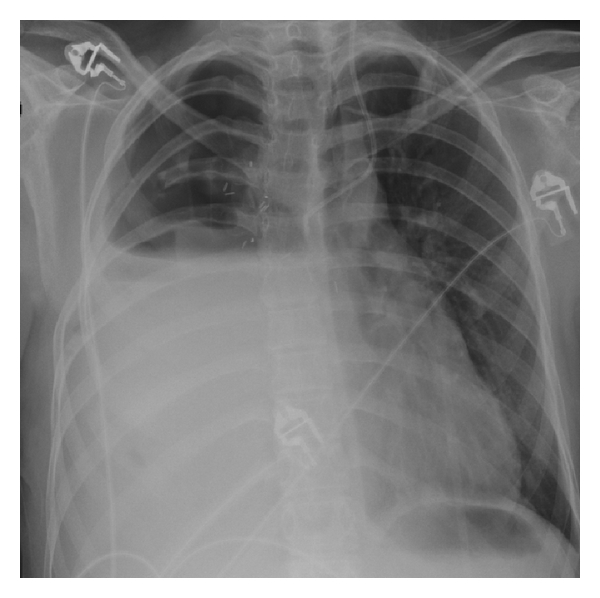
Upright portable chest radiograph obtained 9 days after surgery demonstrates rapid fluid accumulation in the right pneumonectomy space with contralateral shift of mediastinal structures to the left. Both findings are suggestive of excessive fluid volume in the pneumonectomy space.
